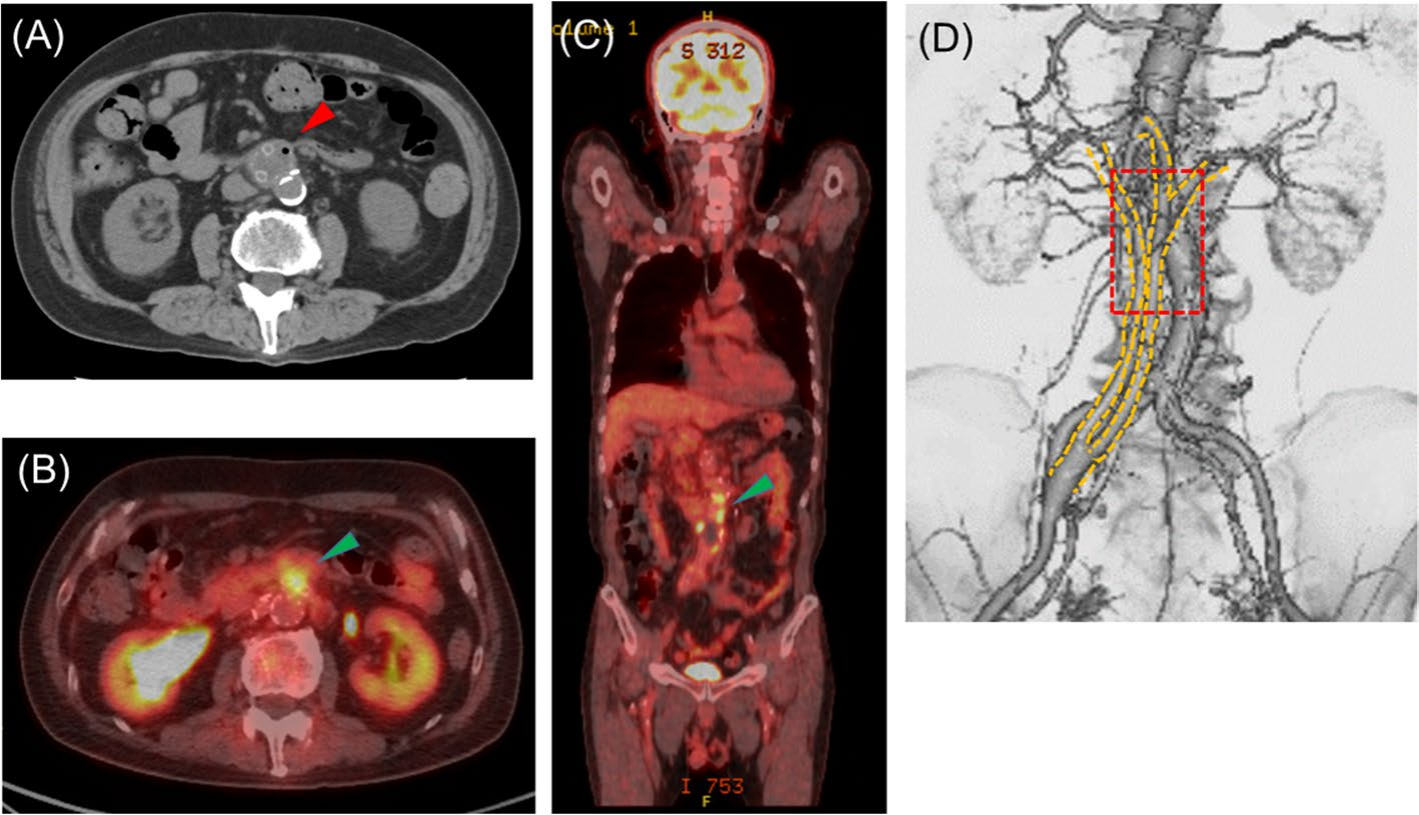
A Abdominal CT before surgery revealed encapsulated fluid and air collection around the grafts (red arrowhead). B, C Representative axial and coronal view of FDG-PET images which revealed focal uptake, SUVmax (maximum standardized uptake value) was 4.7–7.7 (green arrowhead). D We speculated infected area spread in the red-dotted line area by FDG-PET images
